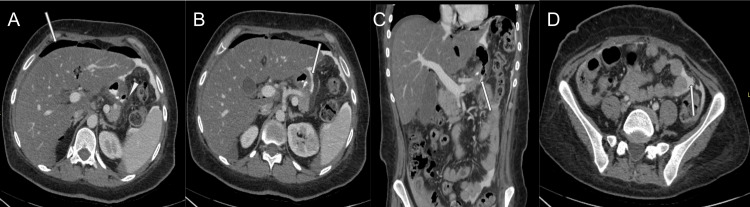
(A) Axial view of the computed tomography (CT) of the abdomen and pelvis demonstrating the gastric wall defect (white arrow head) with leakage of air into the peritoneum and associated pneumoperitoneum in the perihepatic space (white arrow). (B) Axial and (C) coronal CT of the abdomen and pelvis with oral contrast demonstrating contrast leak (white arrow) along the gastric wall defect into the peritoneal space. (D) Axial CT of the abdomen and pelvis demonstrating a leaked contrast material in the pelvis.
